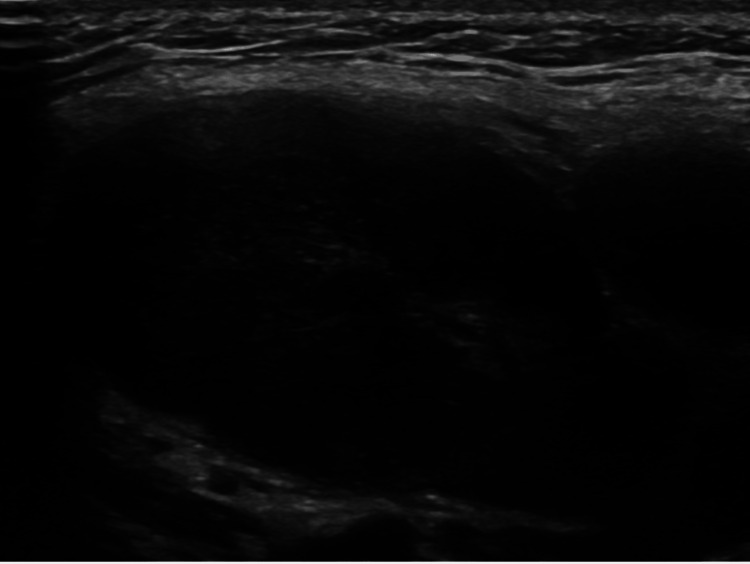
Representative ultrasound image showing swelling on the left side of the patient’s neck Ultrasound image of the left neck revealing a large cystic mass at level II, measuring 2.5 x 2 cm.
